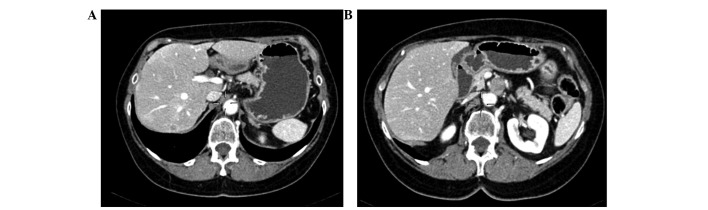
Stable liver and retro-pancreatic metastases during sunitinib treatment. (A) Liver metastasis in segment VIII, showing peripheral enhancement with a central necrotic area on computed tomography. (B) Retro-pancreatic solid lesion exhibiting stable disease with no change in size.
